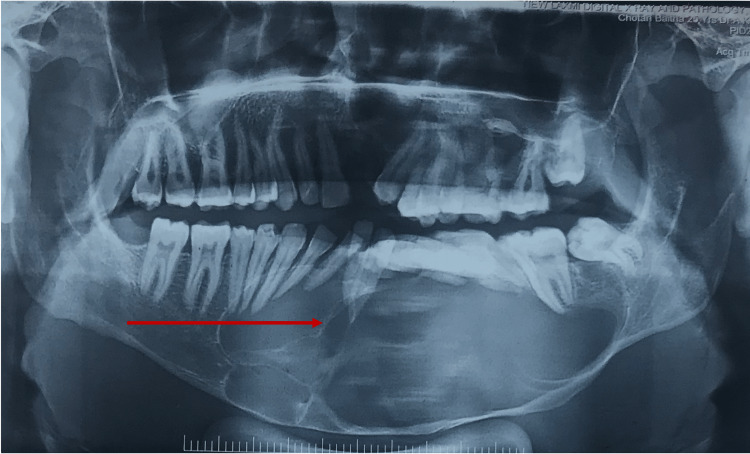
Orthopantomogram showing a large multilocular radiolucent lesion in the mandible with pathological migration of teeth, also external root resorption of anterior mandibular incisor
